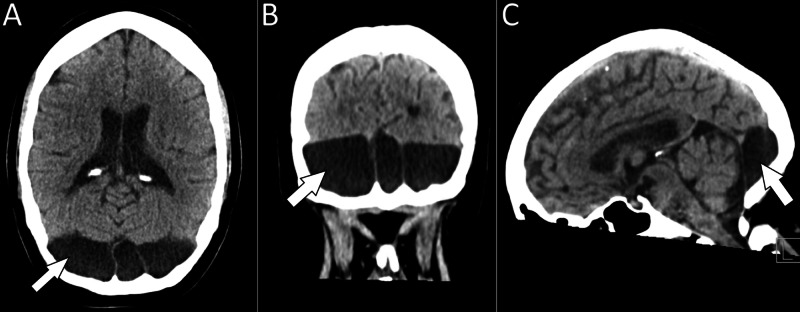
CT of the head shows retrocerebellar hypodensities with septations, consistent with arachnoid cysts bilaterally and in the midline, with mild mass effect on the cerebellum in (A) axial, (B) coronal, and (C) sagittal views.
